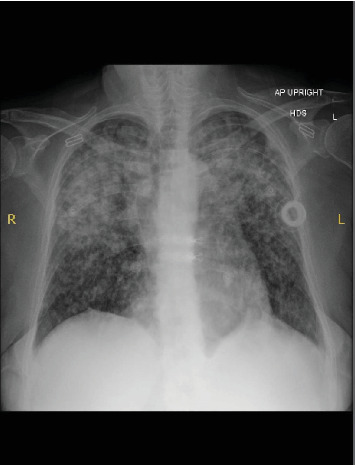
Anterior–posterior chest x-ray showing a bilateral ill-defined nodular pattern.
